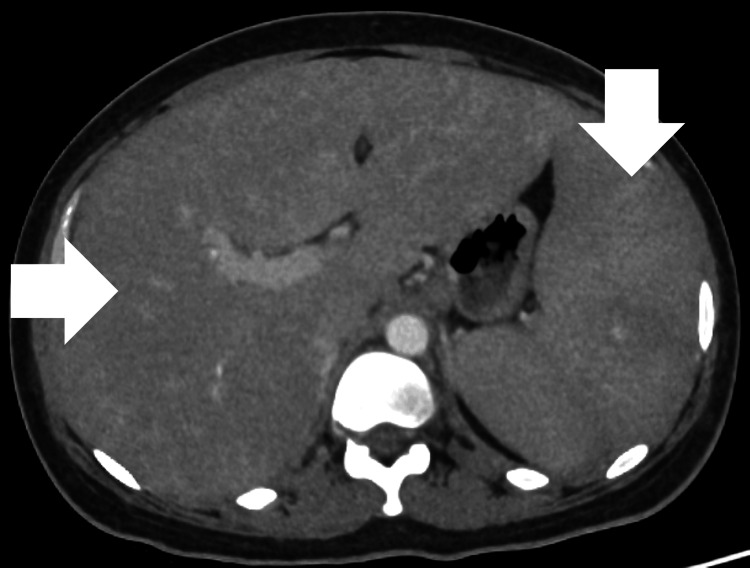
Abdominal CT scan showing hepatosplenomegaly and no other abnormalities.
